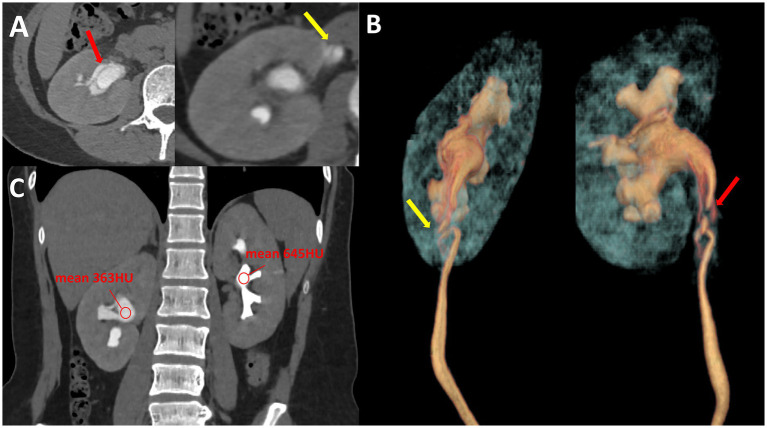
Computed tomography urography: (A) Axial CTU images demonstrate the presence of a double-lumen sign in the renal pelvis (red arrow) and upper ureter (yellow arrow). (B) VR imaging demonstrated tortuous morphology of the proximal ureter (yellow arrow) and the extent of the dissection lesion (red arrow). (C) During the excretory phase, the CT value of the dissected side was significantly lower than that of the contralateral healthy side.
